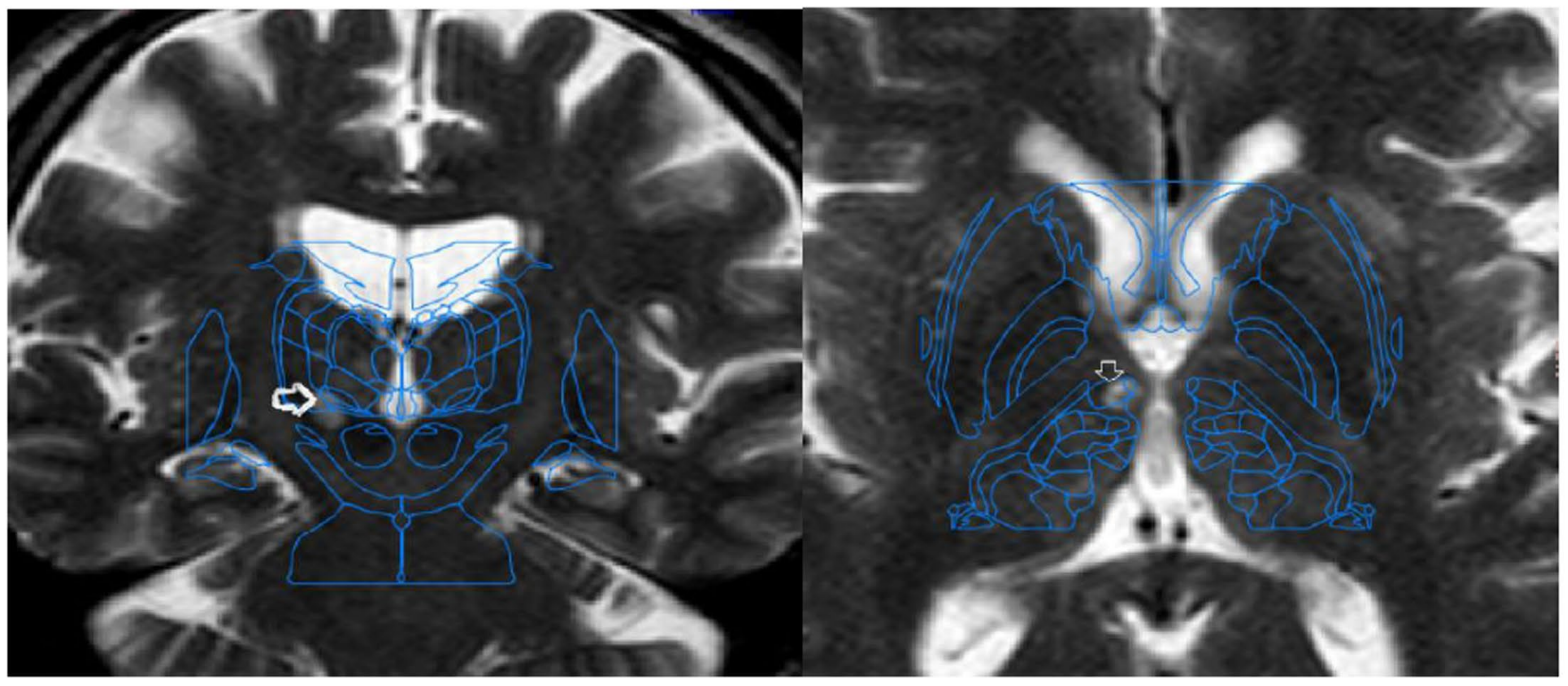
Postoperative coronal and axial T2-weighted MRI images with Schaltenbrand–Wahren atlas fusion, showing microlesions in the caudal zona incerta (left) and fields of Forel (right).
